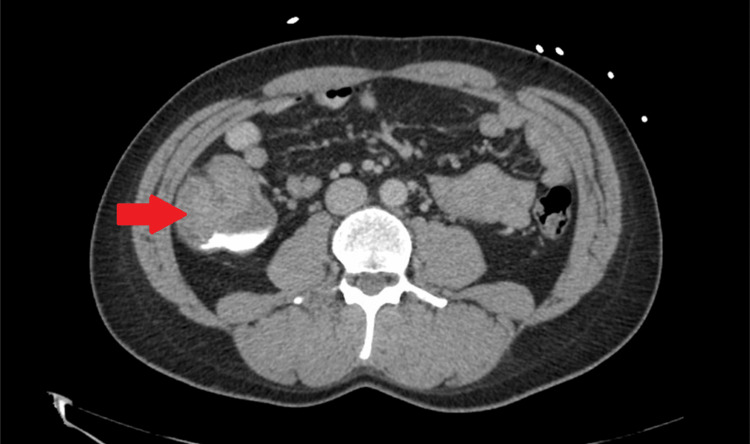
Transverse view of CT scan of the abdomen and pelvis. Red arrow points towards the cecal mass.
